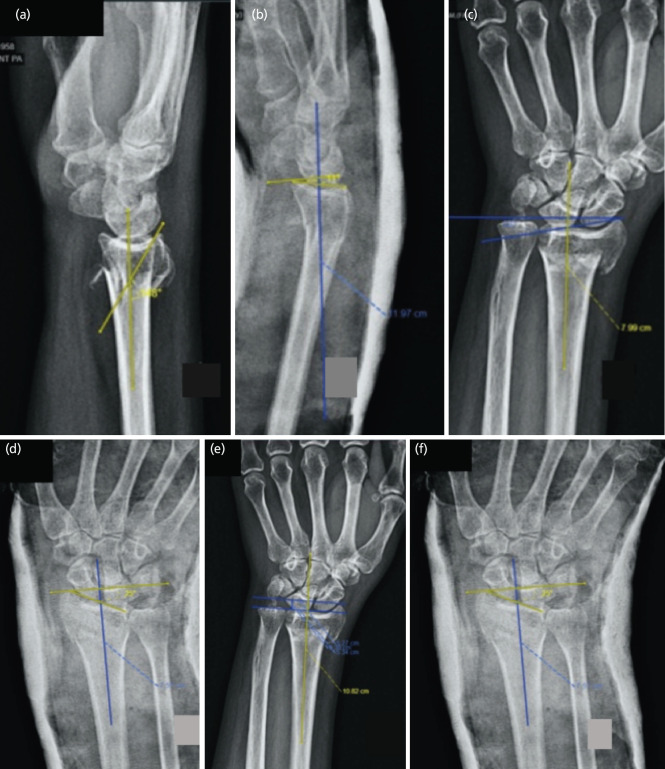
(a – f) Radiographic images, depicting method to measure volar tilt, radial inclination and radial height respectively in a case of distal radius extra-articular fracture.
